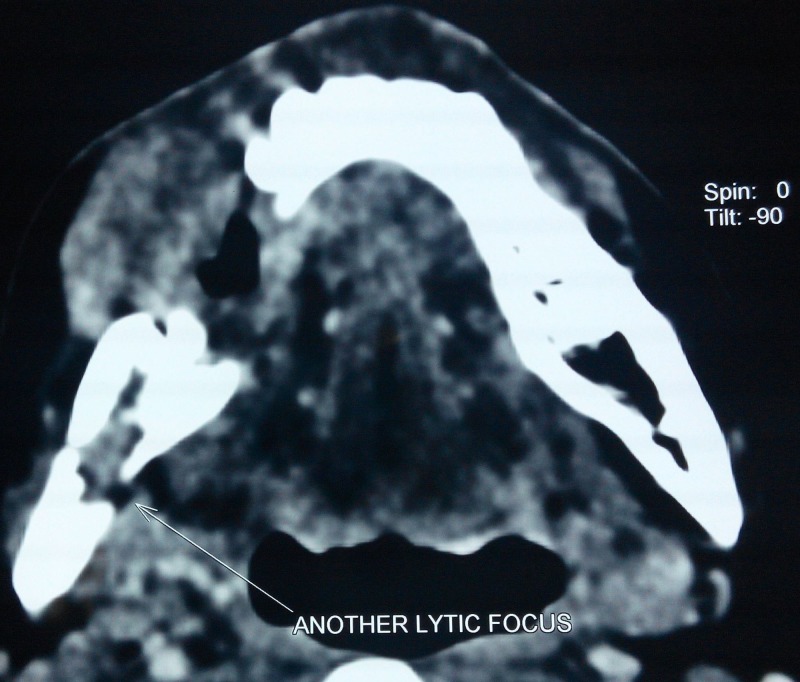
Axial section of computed tomographic image showing another 1 × 1.7 cm ill-defined well-enhancing lytic lesion at right angle of mandible with erosion of lingual cortex.
